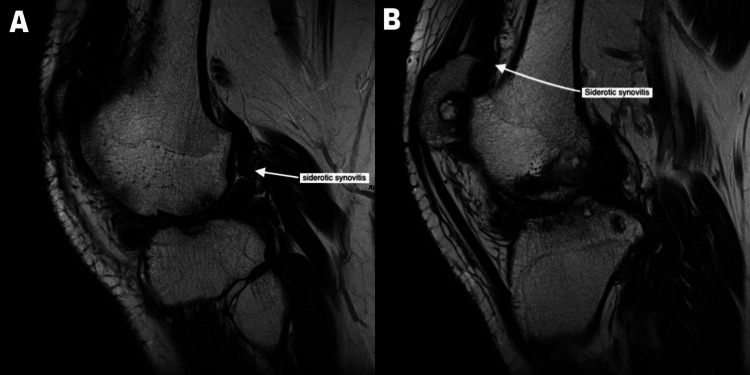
Siderotic synovitis MRI Images showing siderotic synovitis
